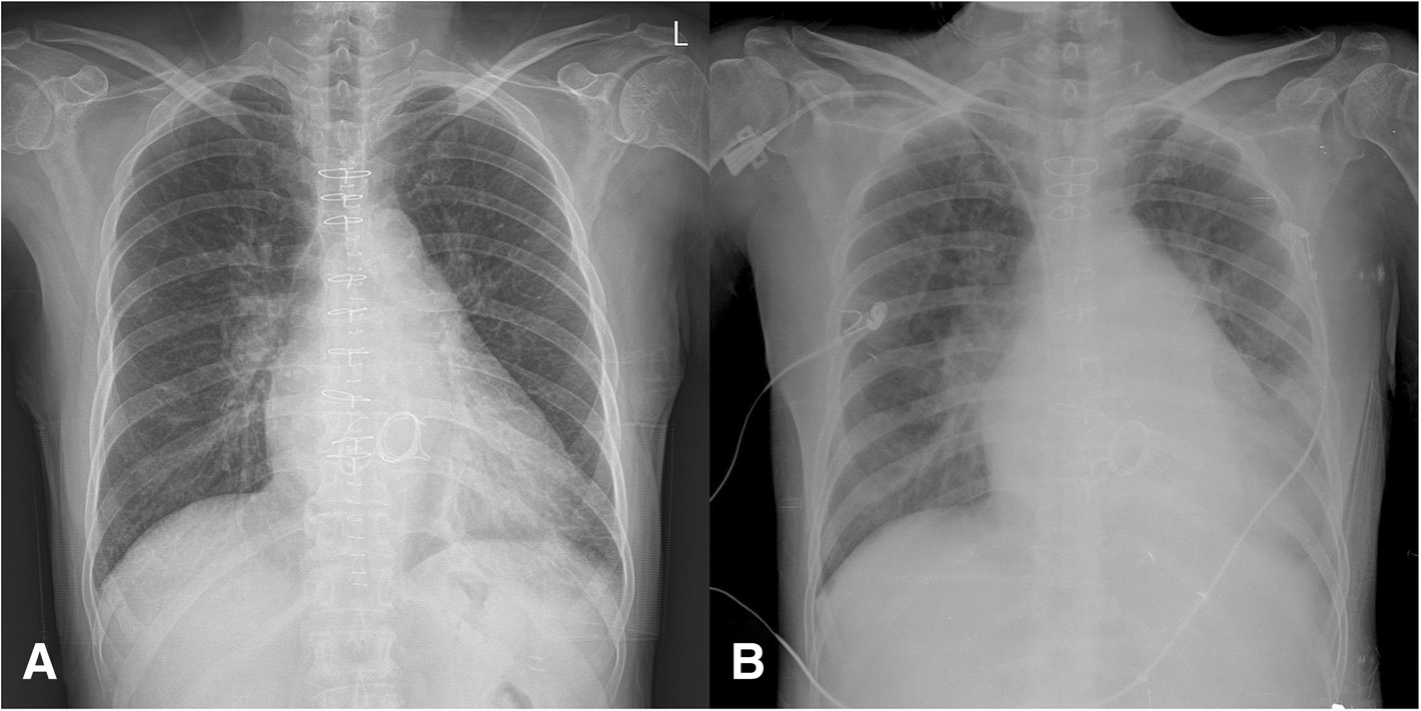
Chest x-ray. Postoperative sixth day chest x-ray (A). On the postoperative 12th day, chest x-ray showed interstitial edema (B).
